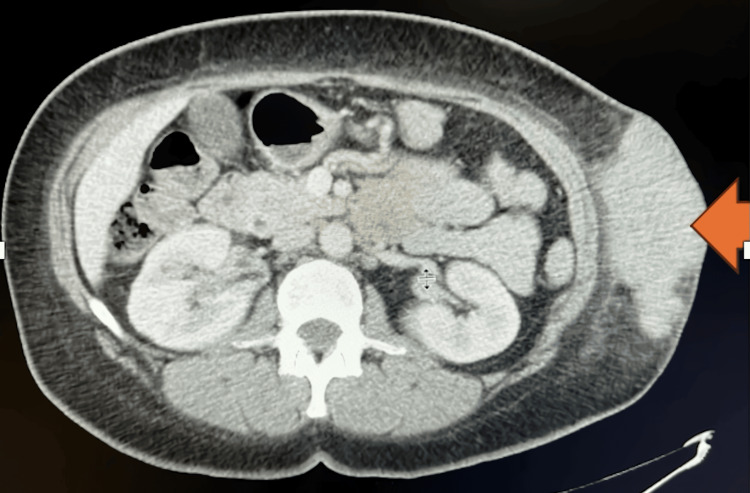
CT of abdomen (coronal view): the orange arrow indicates the soft tissue mass on left flank
